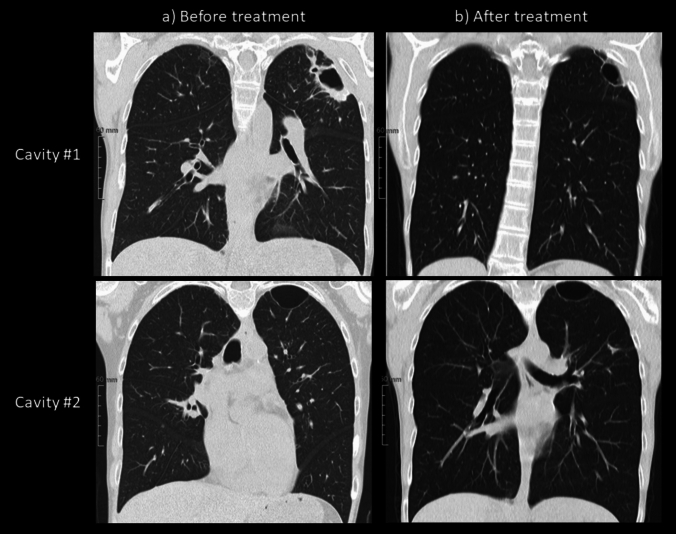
A Chest CT on admission showing two cavitary lesions in the left upper lobe. Only cavity #1 showed signs of inflammation. B Chest CT scan after 12 months of antimicrobial therapy. Cavity #1 showed complete regression of inflammation and significant reduction in size, whereas cavity #2 showed no relevant alteration
